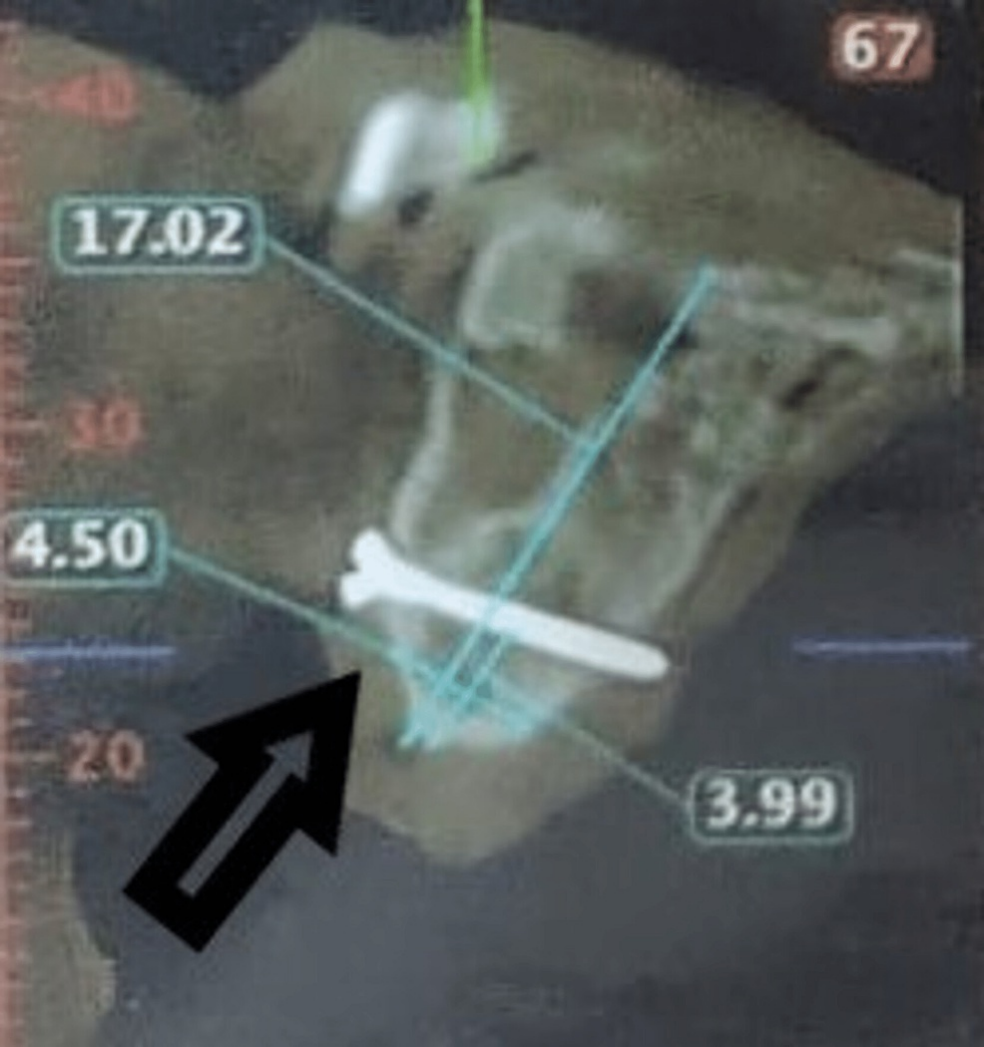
Postoperative CBCT of the patient CBCT: Cone-beam computed tomography
